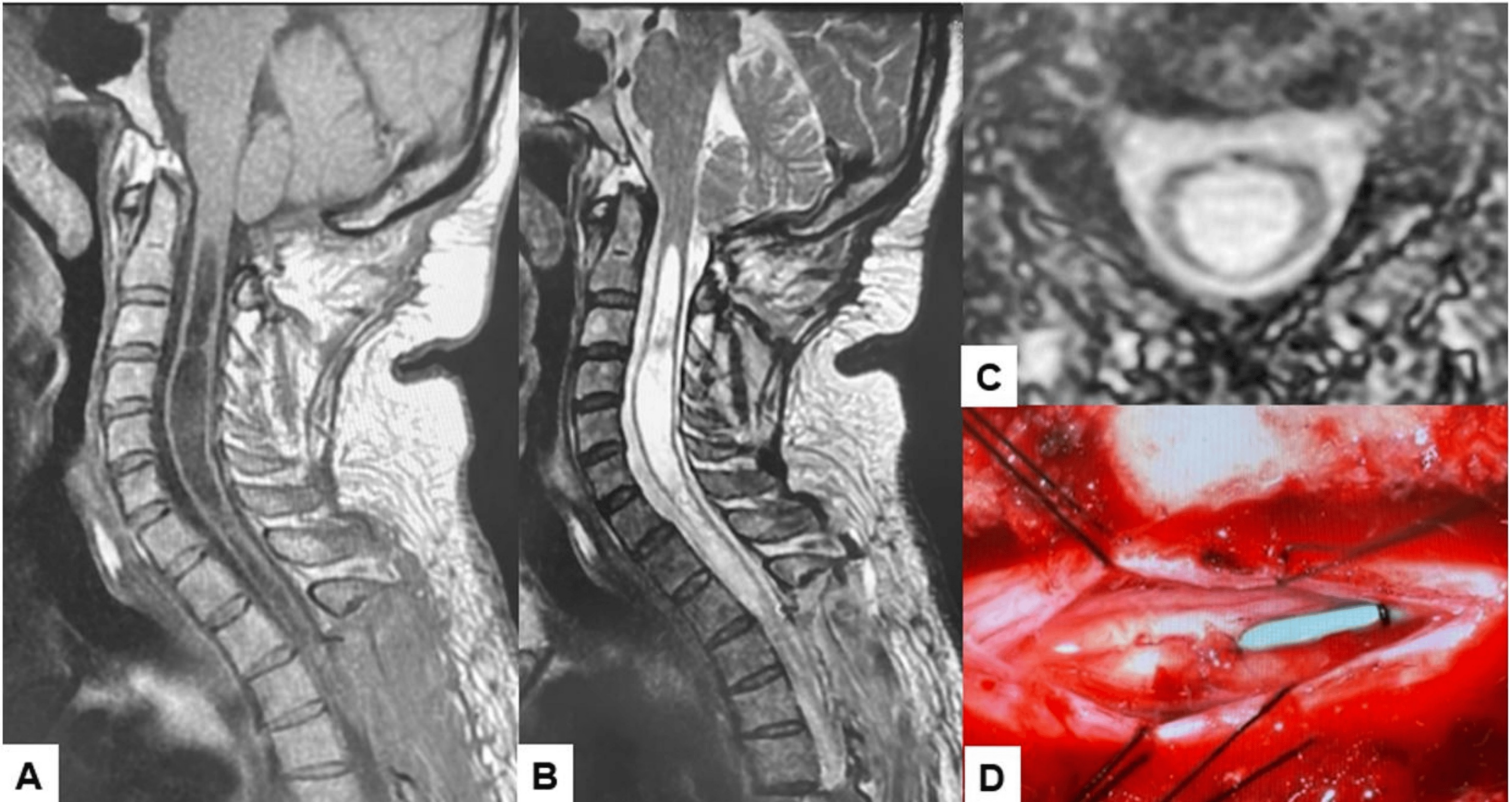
Images after SS shunting: (A) T1 sagittal MRI, (B) T2 sagittal MRI, (C) T2 axial MRI at C4, and (D) SS shunting. The syringomyelia showed a decrease in morphology. MRI: magnetic resonance imaging; SS: syringosubarachnoid
